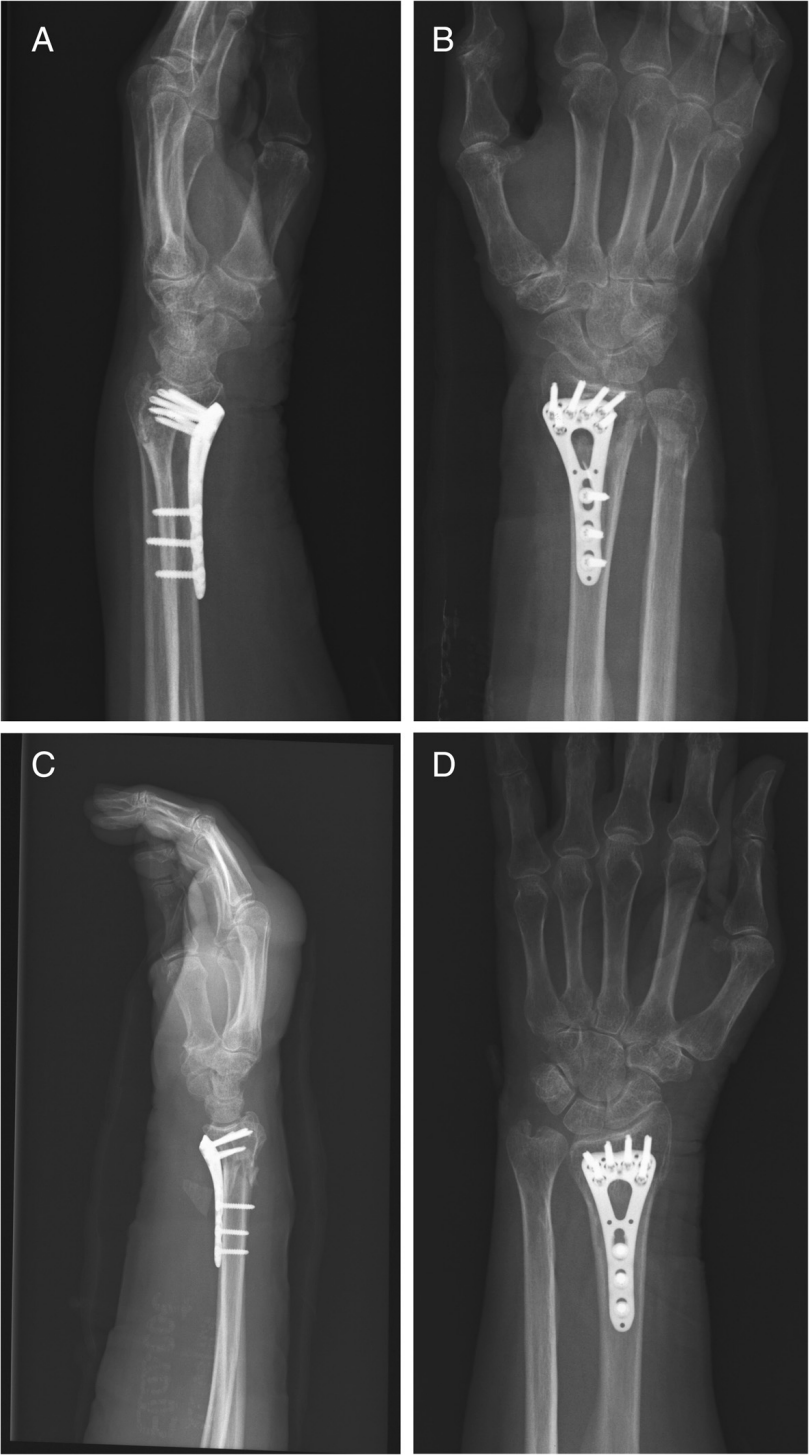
Postoperative radiographs. A: Right side, A-P view. B: Right side, lateral view. C: Left side, A-P view. D: Left side, lateral view.
